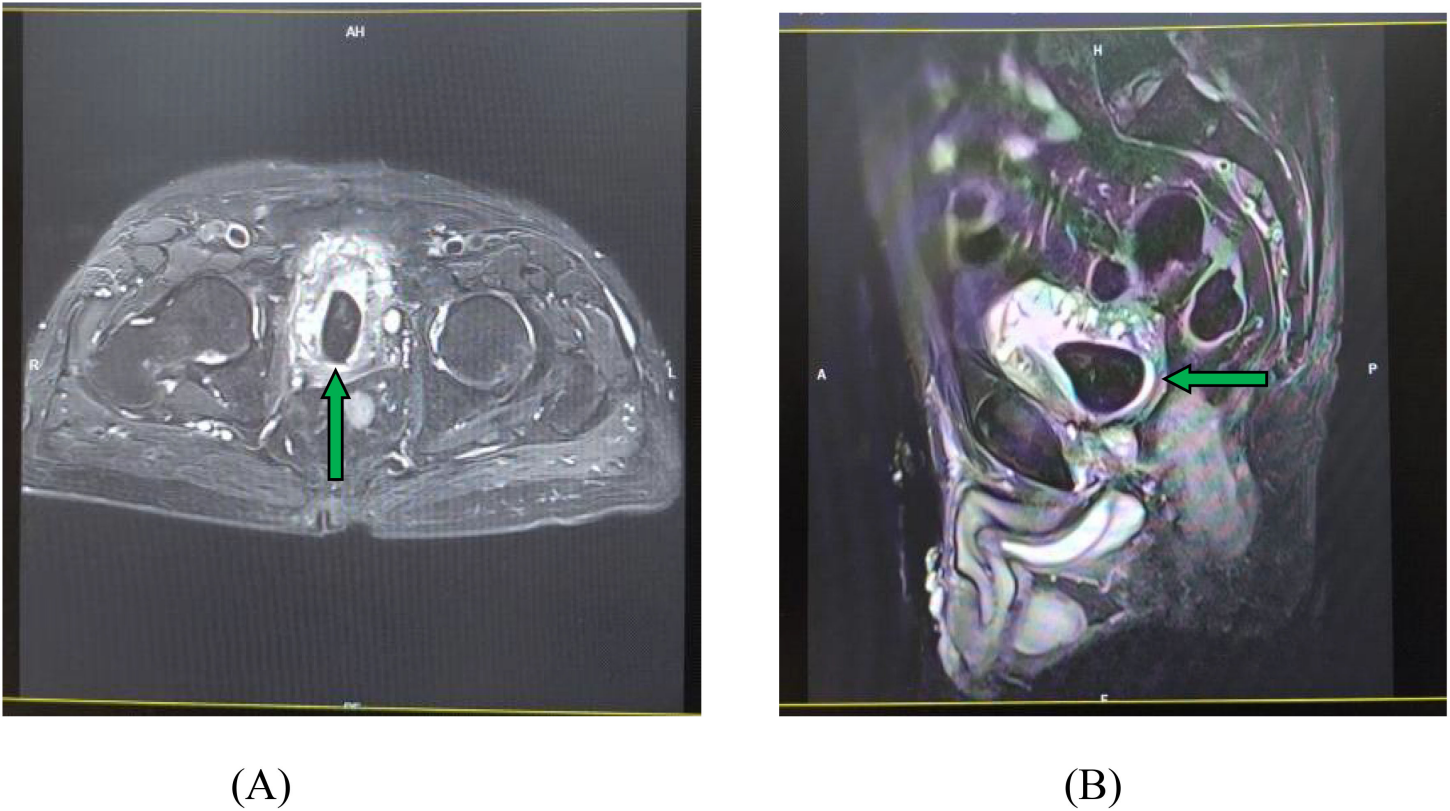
MRI examination of the pelvis showing a large BS. (A) Horizontal view. (B) Sagittal view.
